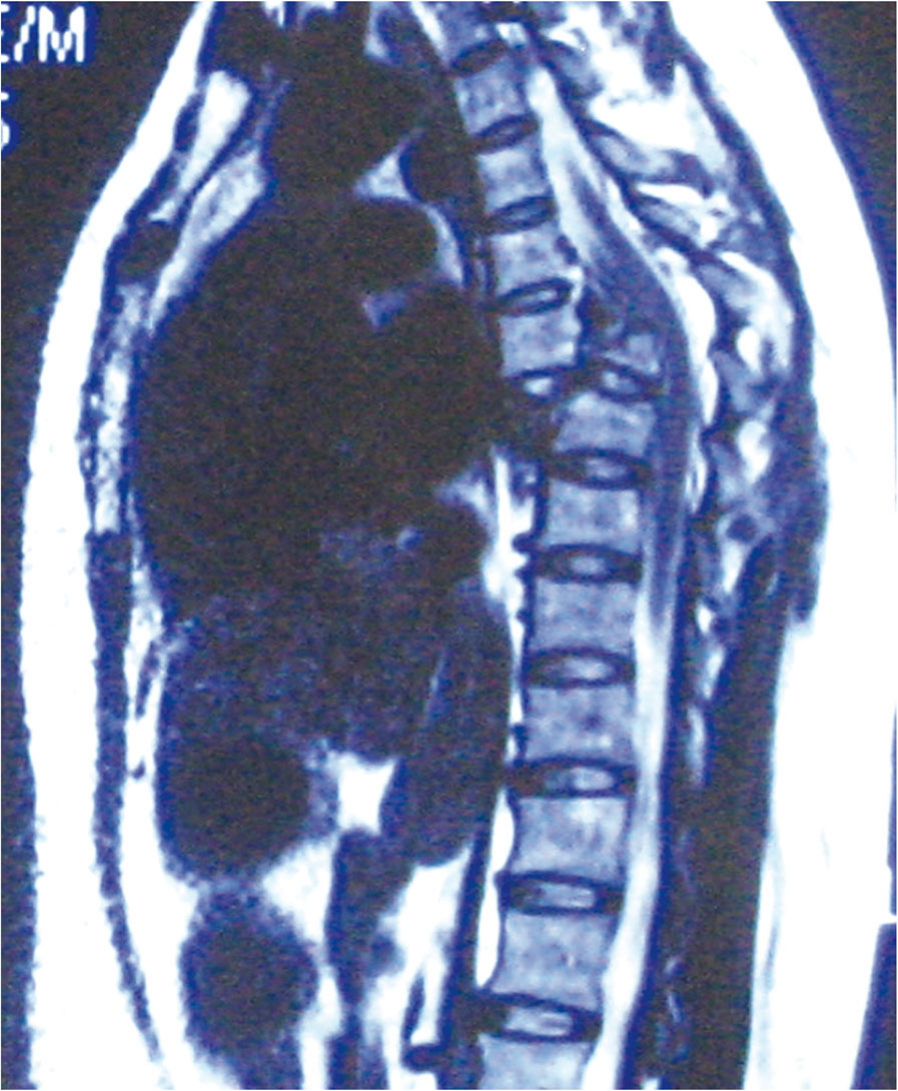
The sagittal MR images revealed that the spinal cord was well decompressed spontaneously
